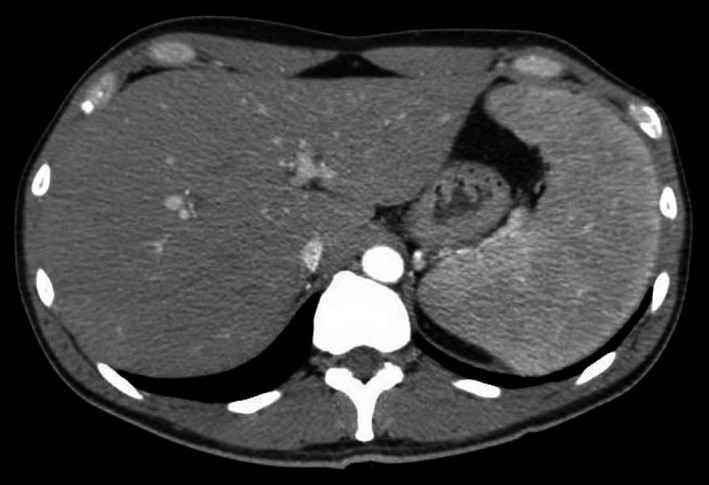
Abdominal and pelvic CT scan with contrast revealing moderate splenomegaly and trace ascites.
